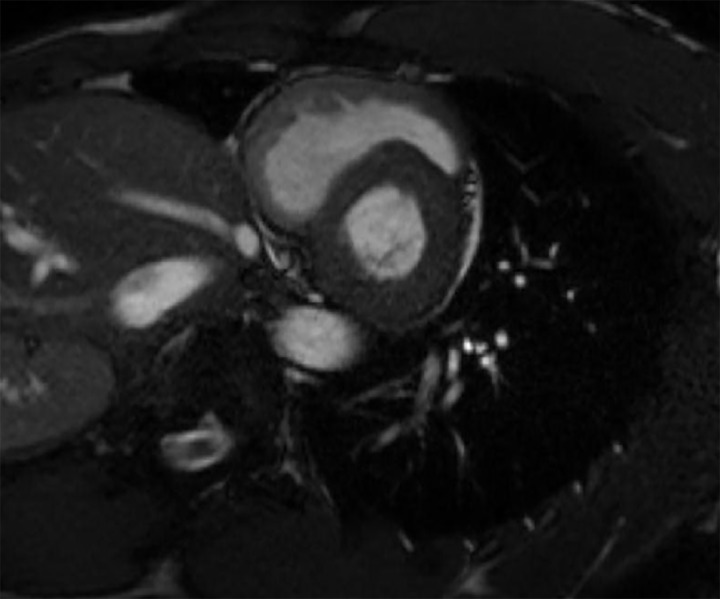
Still image from the cardiac magnetic resonance image of the positive athlete: Demonstrating concentric left ventricular hypertrophy with a septal thickness of 1.3 centimeters most consistent with an athletic heart.
